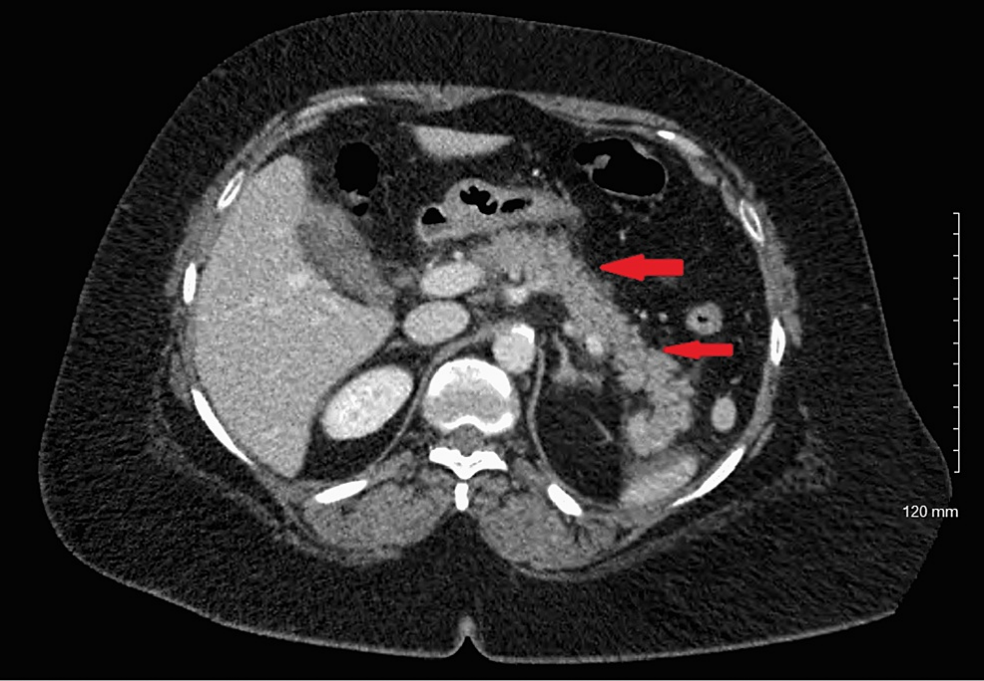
CT scan of the abdomen revealed peripancreatic effusion along the midbody and distal portion
